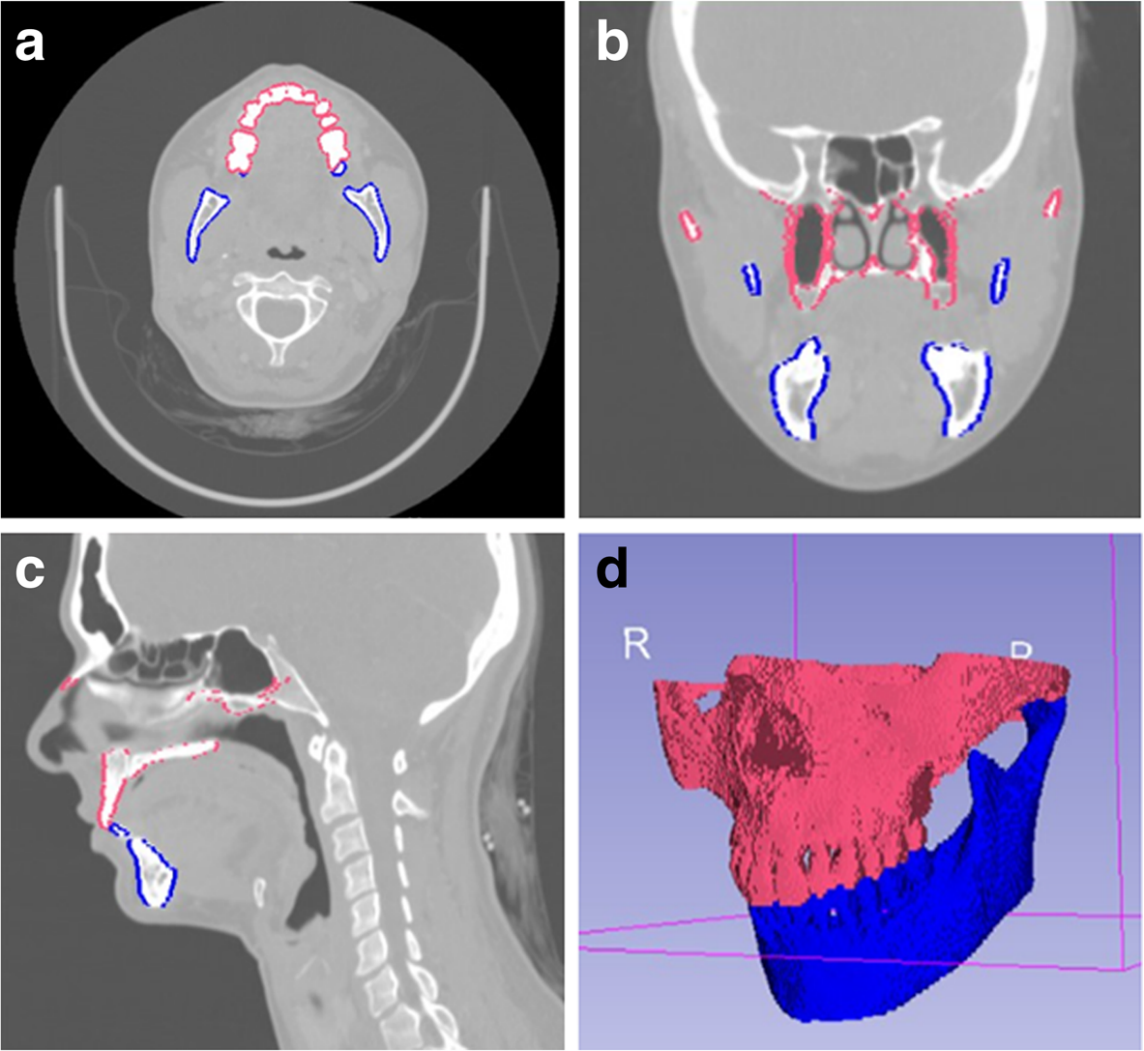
a) Axial, b) coronal and c) sagittal CT images obtained from 3D Slicer depicting the maxillary and mandibular anatomy with d) the corresponding 3D reconstruction
